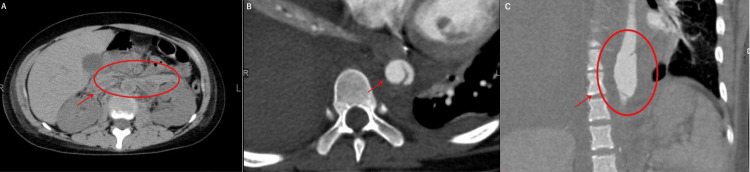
Representative images of rupture of the descending aorta (A) CT scan showing hematoma around the ruptured descending aorta (red arrow and red circle). (B) Axial view of contrast-enhanced CT scan showing dissection cavity (red arrow). (C) Coronal view of contrast-enhanced CT scan showing thoracic aortic aneurysm (red arrow and red circle).
